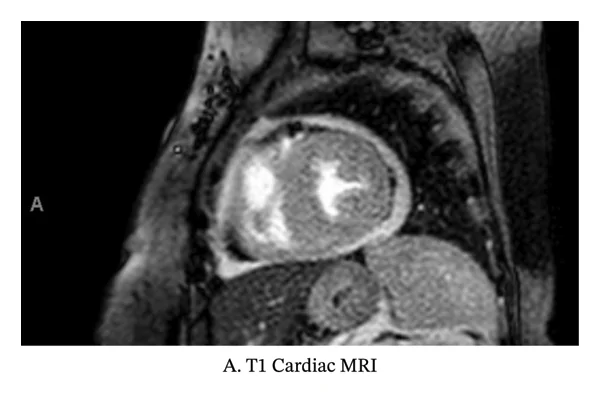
Cardiac MRI showing elevated native T1 suggestive of diffuse myocardial fibrosis.
